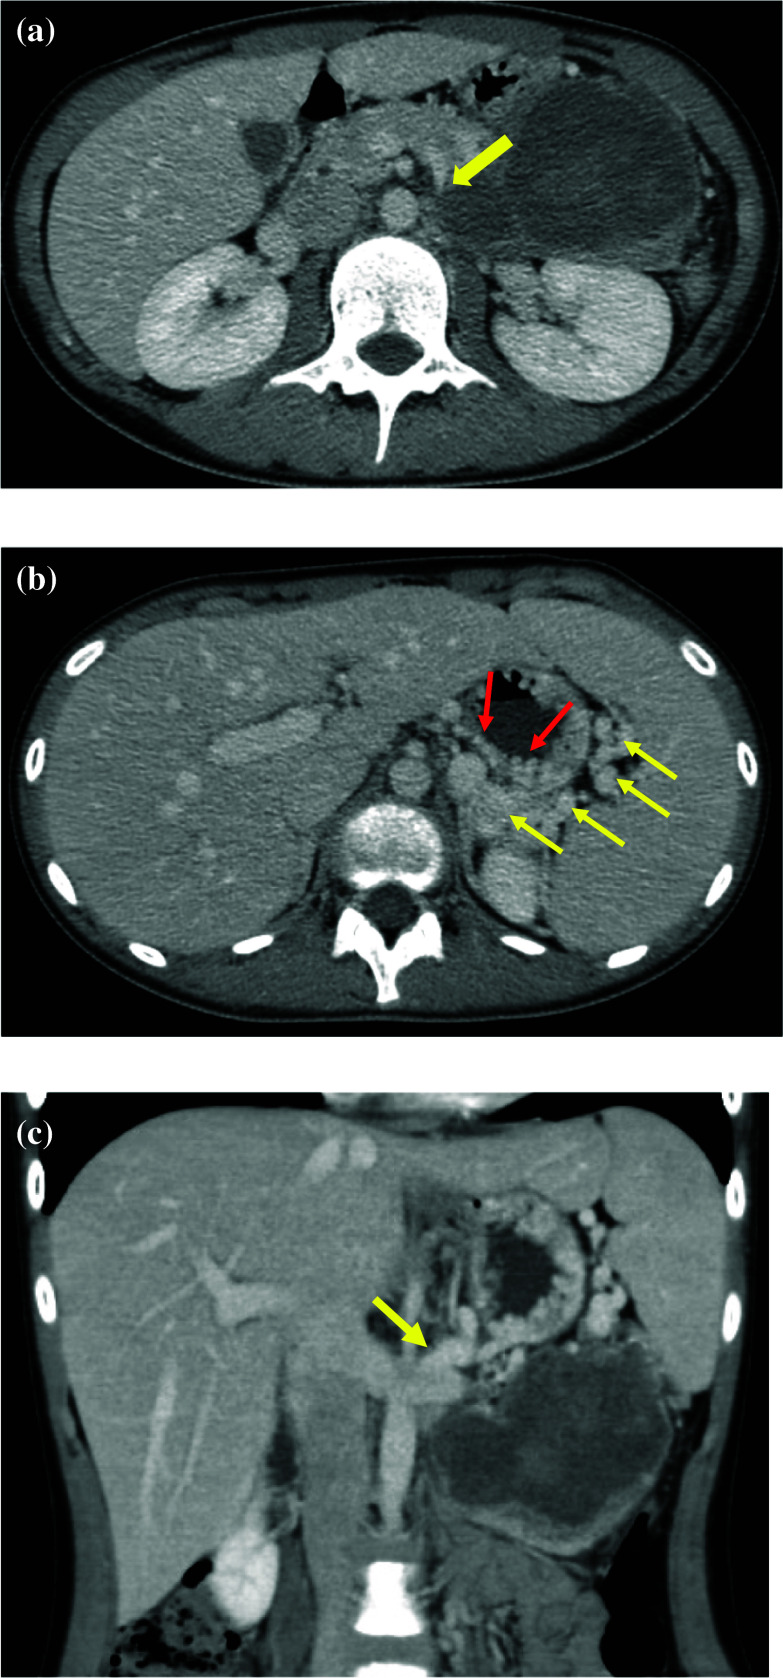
a Enhanced CT. a Shows that the splenic vein was narrowed like as beak due to compression and stretch by the tumor. b Enhanced CT. Collateral pathways developed through a short gastric vein and the left gastric vein (yellow arrow). Varices were developed in the gastric wall (red arrow). c Enhanced CT. A large spleno-renal shunt was developed (yellow arrow)
